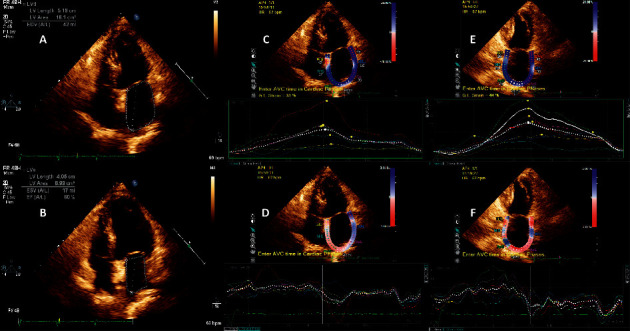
Cardiac function measured by echocardiography and speckle tracking echocardiography. (a, b) Measurement of maximum LA volume and minimum LA volume by the single-plane Simpson's method through an apical four-chamber view. (c, d) Atrial strain and strain rate measured in an apical four-chamber view. (e, f) Atrial strain and strain rate measured in an apical two-chamber view.
